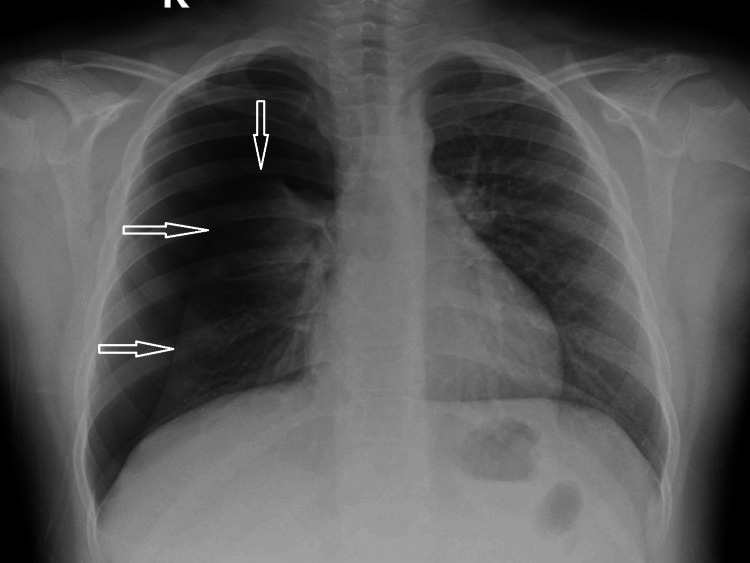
Chest radiograph showing recurrent ipsilateral pneumothorax Chest X-ray demonstrating recurrent right-sided pneumothorax. Hollow white arrows indicate the margins of the collapsed lung.
